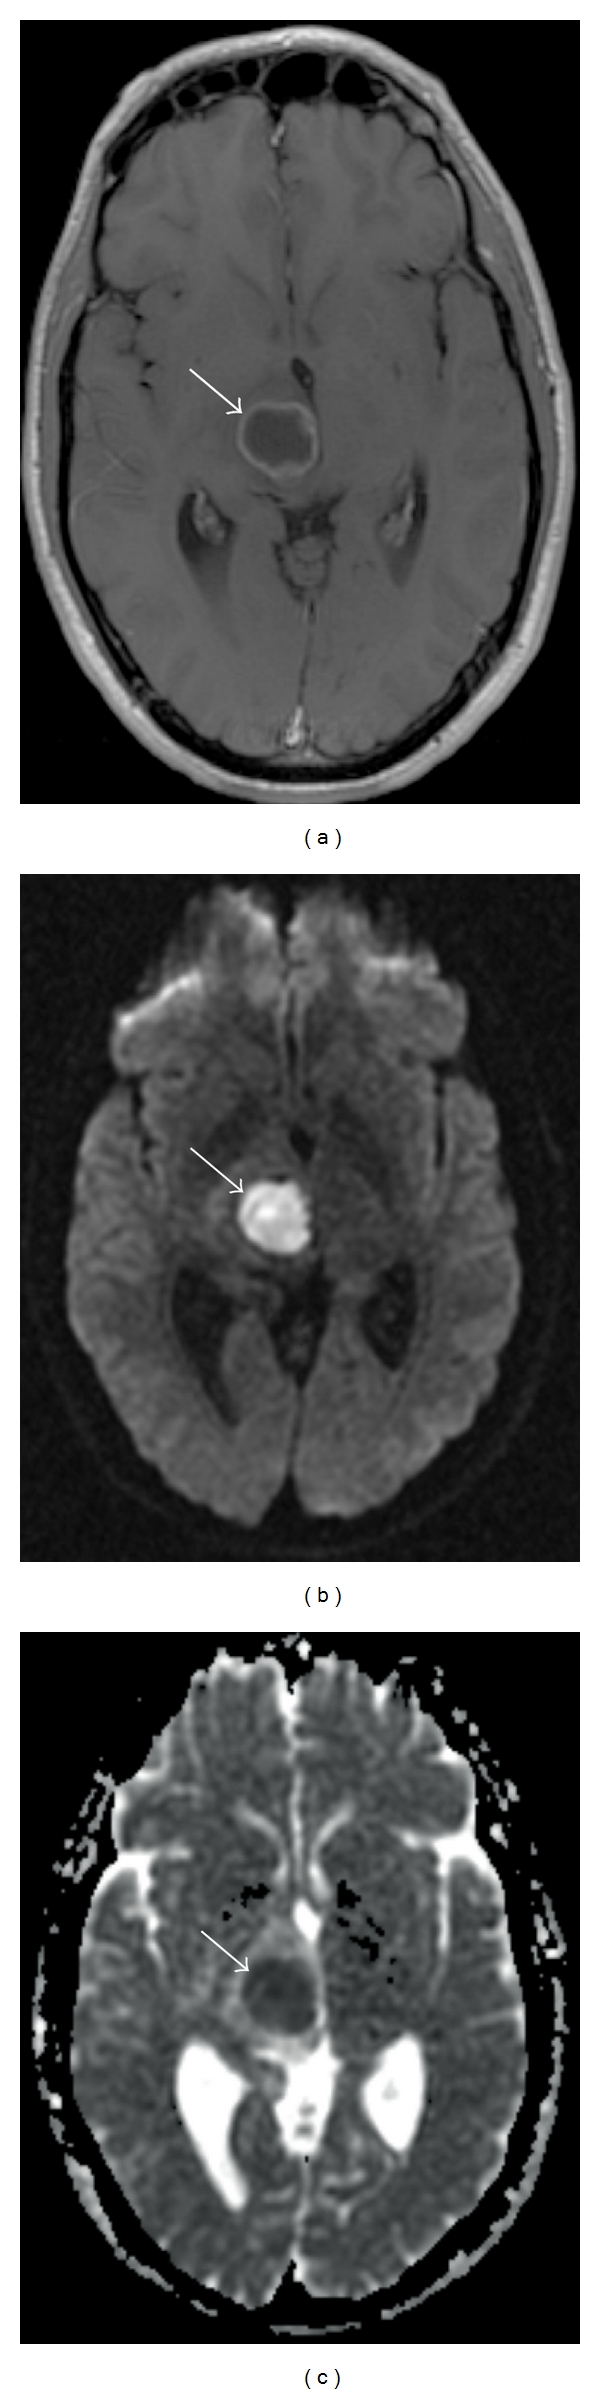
A 19-year-old man presented with diplopia and left-sided weakness, and a right thalamic abscess. (a) Axial T1 postcontrast. A ring enhancing mass is present in the right thalamus (arrow). (b) DWI sequence. Signal hyperintensity is present in the lesion (arrow). (c) ADC map. Signal hypointensity in the lesion (arrow) confirms decreased diffusion. In the appropriate clinical context, this constellation of findings is consistent with a pyogenic abscess.
